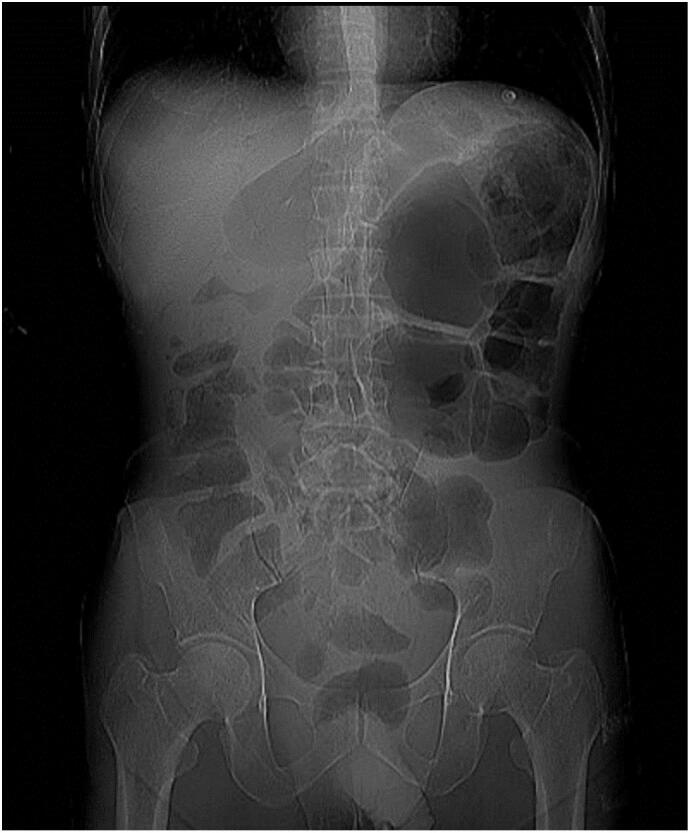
On the plain abdominal X-ray alone, there was no definitive visible evidence of a cholecystoduodenocolic or gastrocolic fistula.
